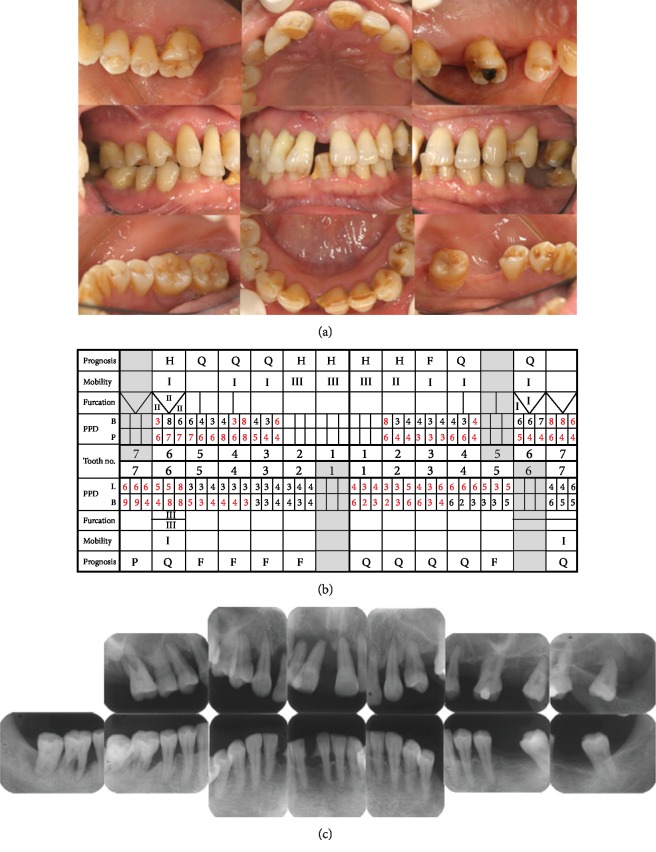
(a) Pretreatment intraoral photographs (April 2004). Generalized inflammation is observed, particularly between tooth #11 and tooth #12, where a fistula is present. (b) Pretreatment periodontal chart. Teeth #11, #12, #16, #21, and #22 were evaluated as “hopeless”; #46 and #47 were “poor” (PPD: periodontal probing depth; G: good; F: fair; Q: questionable; P: poor; H: hopeless, red: bleeding). (c) Pretreatment radiographs. Thirteen dental X-ray films show severe bone loss in the maxillary anterior and mandibular right molar regions.
